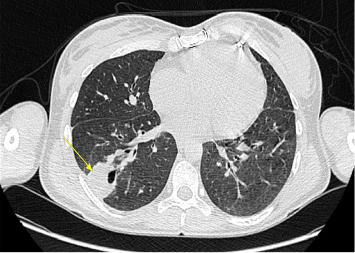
CT findings in keeping with the plain film appearances, demonstrating a cavity within the right lower lobe with the luminal surface irregular relatively extensive adjacent consolidation (yellow arrow).
